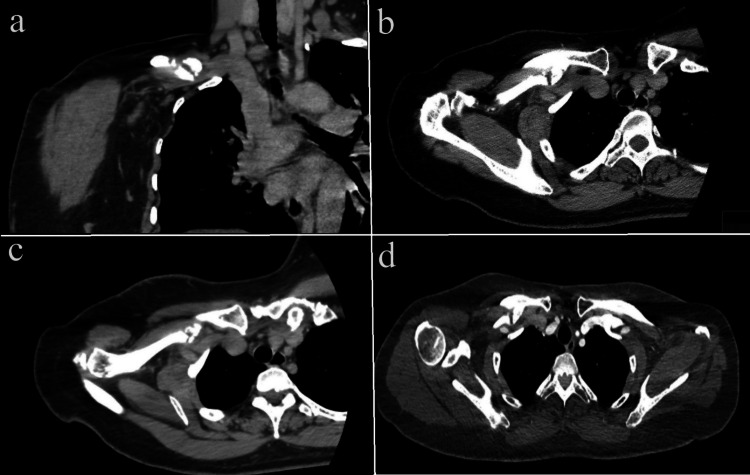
Case 1: Pre-operative shoulder CT scan depicting nonunion and abundant callus at the fracture site, with the axillary vein closely situated a (coronal cut) and b and c (axial cut) highlight these features. d displays the CT angiogram, indicating compression of the axillary vein by the lateral segment at the fracture site.
